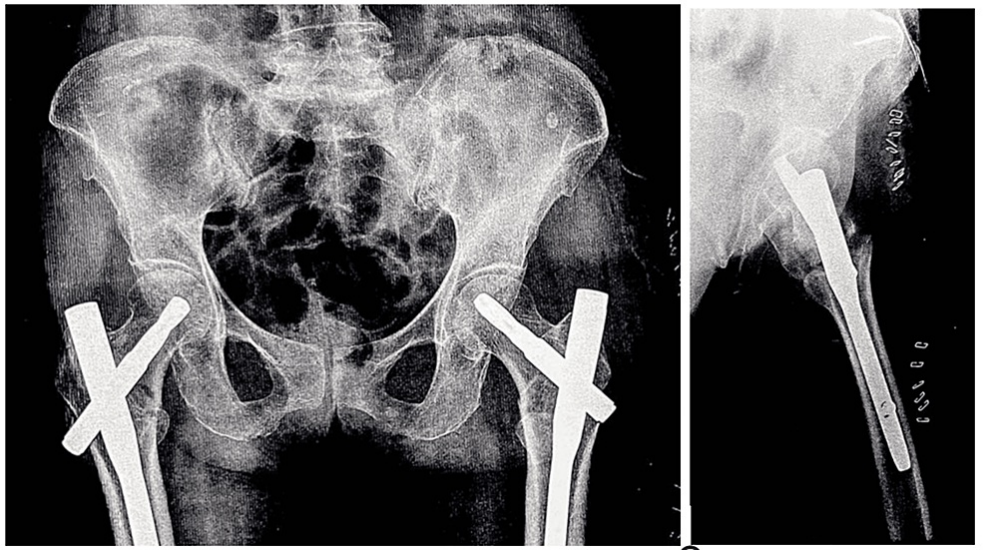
Plain radiograph of the pelvis with both hips showing operated cases of bilateral intertrochanteric fracture with in situ PFNA2 PFNA2: proximal femoral nail anti-rotation Asia
